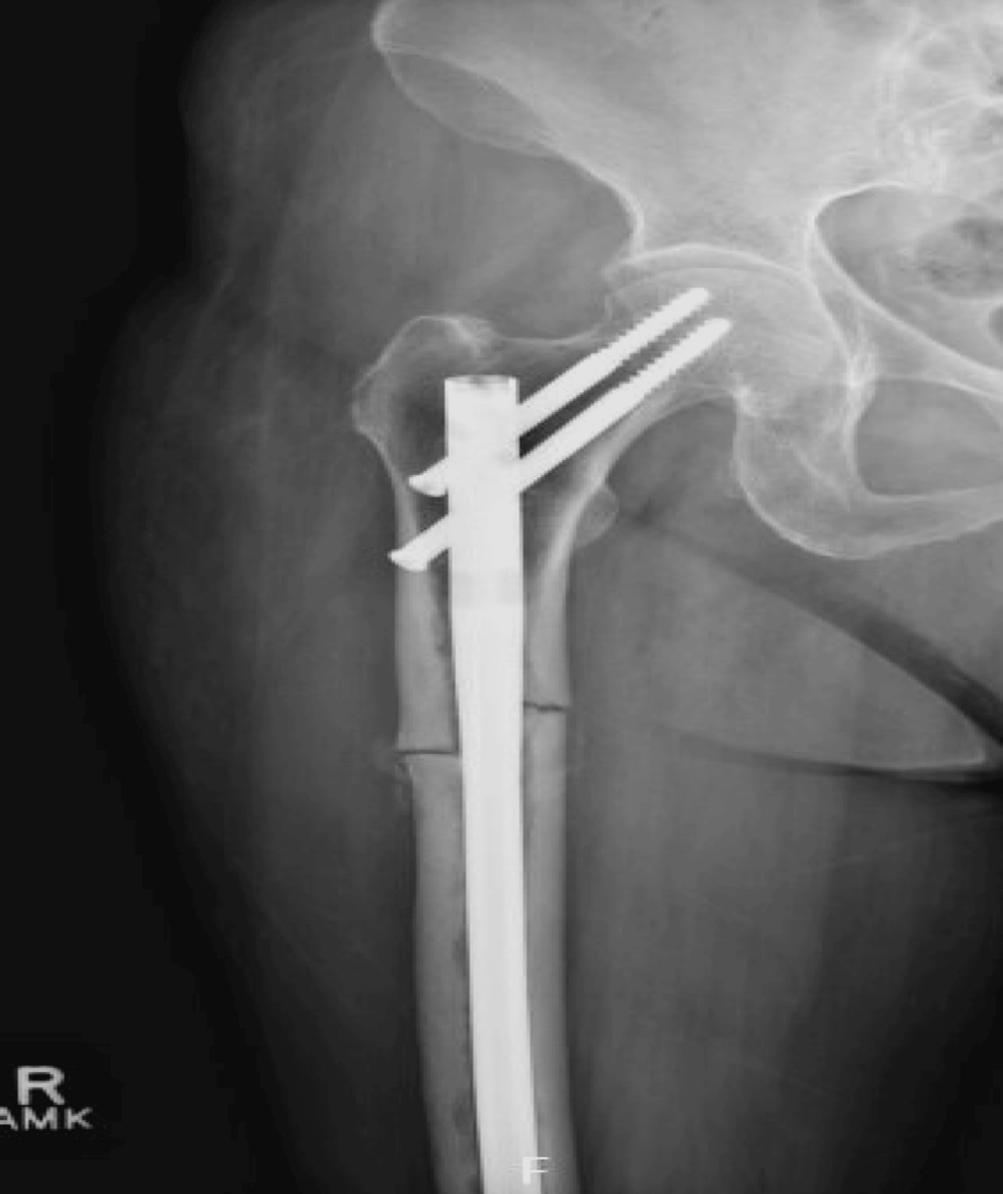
Postoperative radiograph showing intramedullary nail fixation of the subtrochanteric fracture. R: right, F: fracture, AMK: anteromedial knee.
